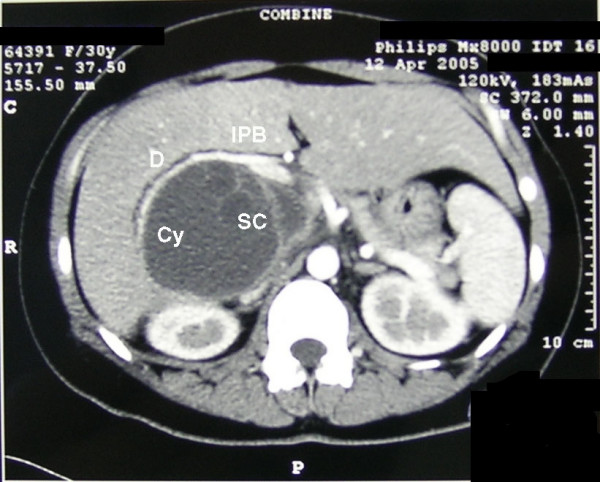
Contrast-enhanced CT scan. Cy: cyst; D: fissured cyst wall; IPB: intra-hepatic portal branch to segments VI and VII; SC: small cyst.
